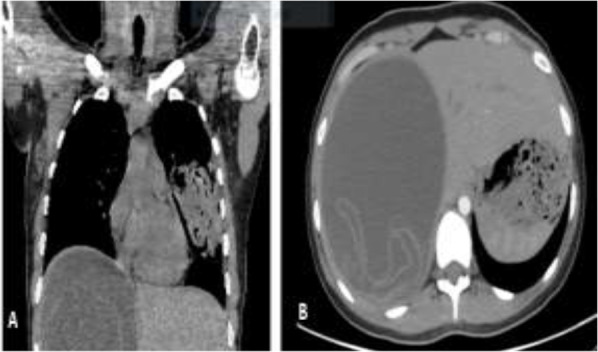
A, Coronal CT scan; Hydatid cysts in liver and the left lung. B, Axial CT scan; large hydatid cyst in the liver
